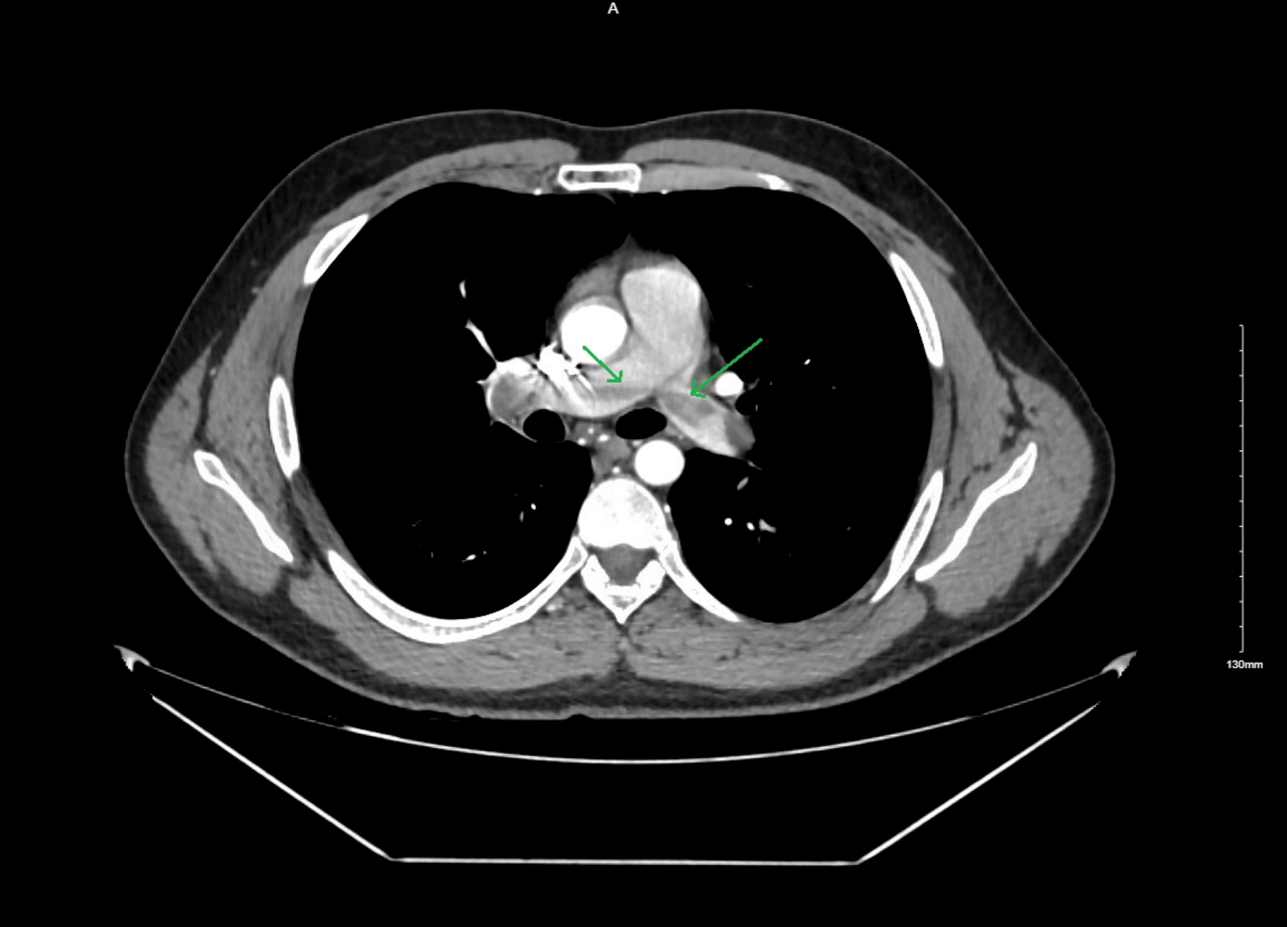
Computed tomography angiography (CTA) of the chest showing a saddle pulmonary embolism. A large thrombus is visualized at the bifurcation of the main pulmonary artery with extension into both the right and left pulmonary arteries (green arrows).
